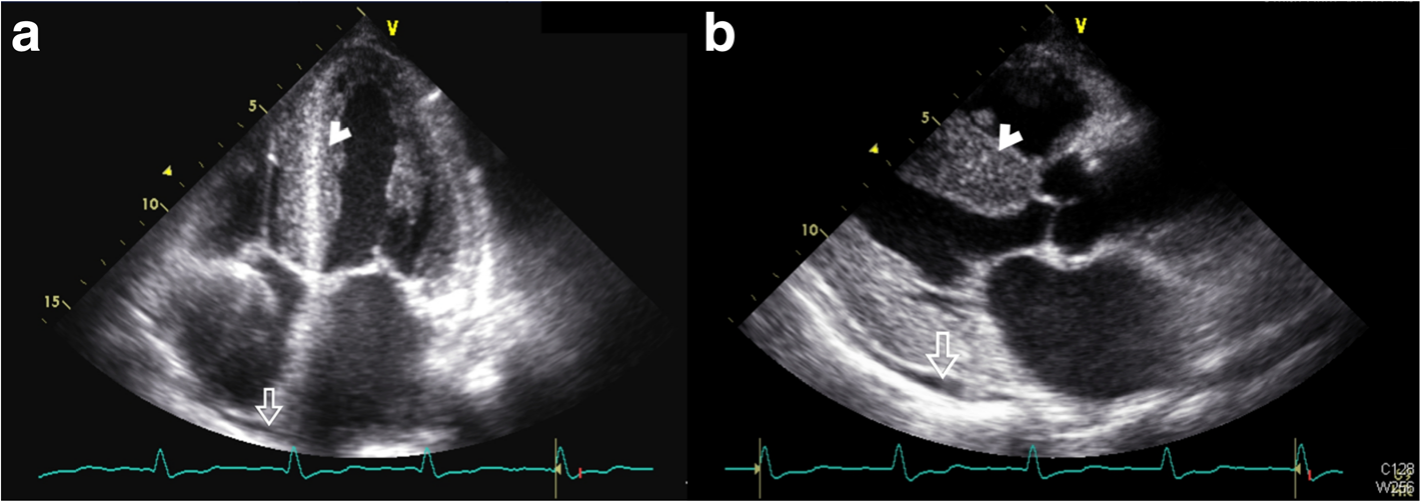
a In an apical 4-chamber view, biventricular wall thickening, granular sparkling (arrowhead), thickening of the mitral and tricuspid valves, and a trivial amount of pericardial effusion next to the right atrium are visible in the systolic phase (arrow) (patient 6, age 65). b In a parasternal long-axis view, a left atrium dilatation and a very thick granular myocardium (arrowhead) with some pericardial effusion (arrow) at the baso-posterior wall are evident (patient 6, age 65)
